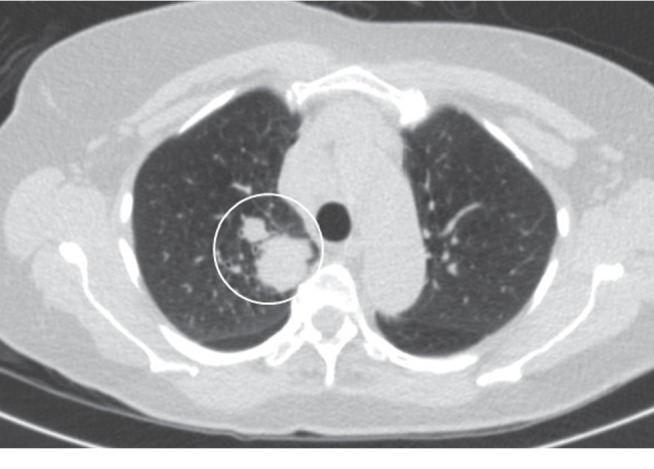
CT scan of the chest showing a 4.2 cm lobulated pleural-based mass in the right upper lobe with enlarged hilar lymph nodes suggestive of nodal metastasis.
